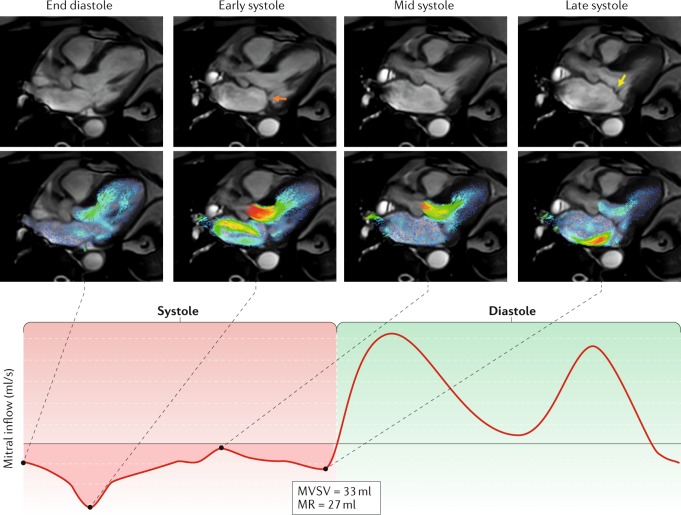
Time-resolved velocity vector visualization with CMR of dynamically changing MR jet. The top row shows cardiovascular magnetic resonance imaging (CMR) of three-chamber cines acquired during four phases of the cardiac cycle: end diastole, early systole, mid systole and late systole. The second row of images shows velocity vectors in the left atrium and the left ventricle superimposed on the three-chamber cines. These images allow the visualization of the A2–P2 scallops, demonstrating early systolic prolapse of the P2 scallop (orange arrow) resulting in a mitral regurgitation (MR) jet directed towards the medial interatrial septum, which settles in mid systole. A late-systolic, posteriorly directed MR jet (yellow arrow) can be appreciated as a result of A2 prolapse. The MR volume is quantified in the lower panel. This example highlights how cine CMR and augmented streamline visualization of the 4D-flow CMR can help to make a more dynamic pathophysiological diagnosis of the cause of MR. MVSV, mitral valve stroke volume.
